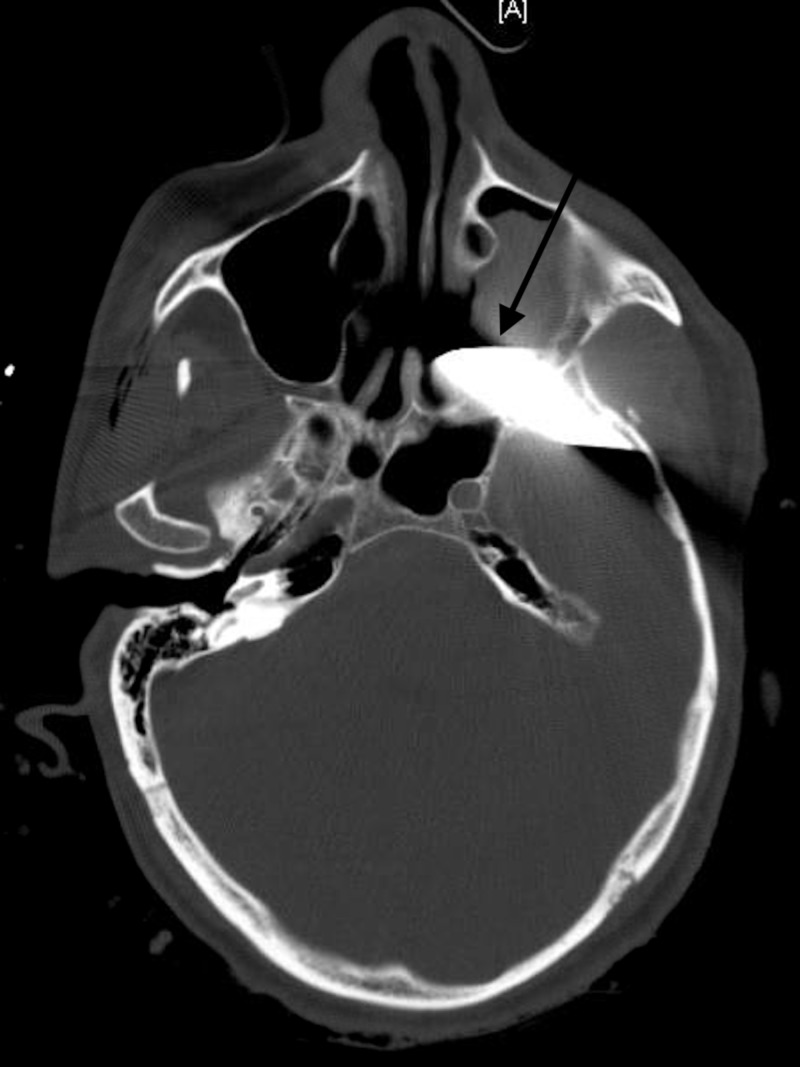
CT of the head The image shows knife penetrating the left temporal bone, left sphenoid sinus, left pterygopalatine fossa, and left maxillary sinus (black arrow) CT: computed tomography
